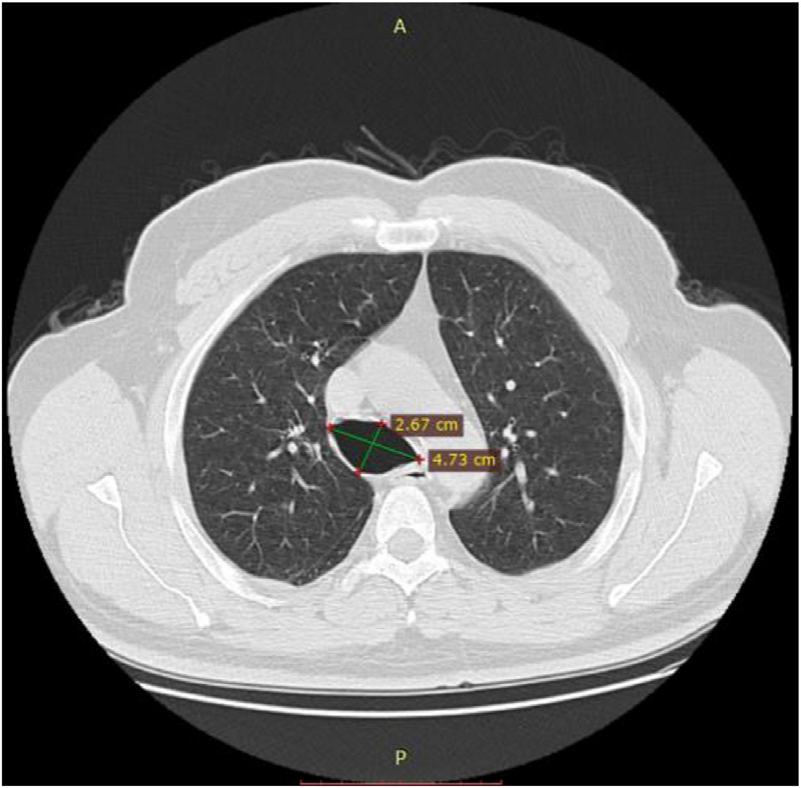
Thoracic CT scan: axial section through the parenchymal window passing through the trachea. The transverse diameter of the trachea is 47.3 mm; the sagittal diameter is 26.7 mm.
